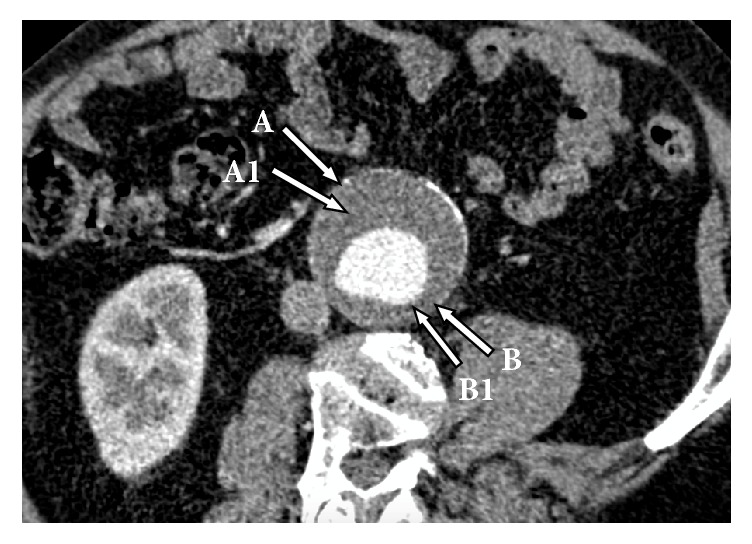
Typical computed tomography image of patient suffering from an abdominal aortic aneurysm, demonstrating the sites of sampling: A, wall adjacent to thick thrombus segment; A1, thick thrombus section; B, wall underlying thin thrombus section; B1, thin thrombus section.
